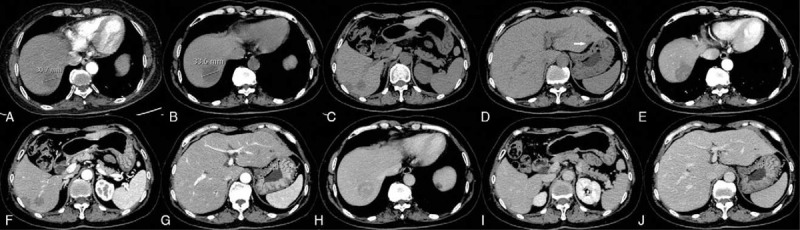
Computed tomography (CT). (A–C) Three rounded, hypodense lesions located in right liver lobe (diameter: 3 cm, 1 cm, 1.1 cm). (D) One oval-shaped hypodense lesion of 0.6 cm in the left lateral lobe of the liver. (E–G) Arterial-phase images showing patchy enhancement in the lesions and (H–J) hyperdense to isodense appearance in the portal phase. Please note the increase in size of the largest lesion from 30.7 mm (A) to 33.6 mm (B) in 2 months.
